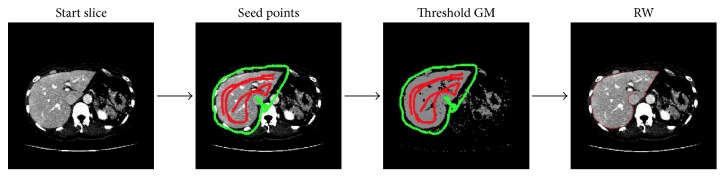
Interactive segmentation of the start slice in CT image.
